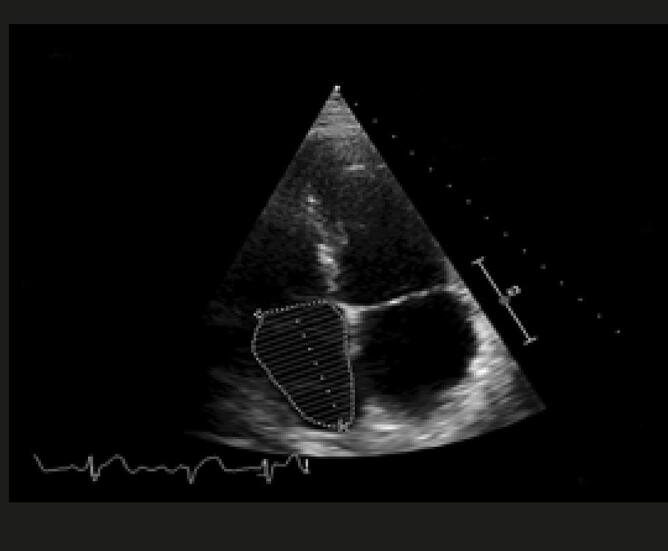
O parâmetro recomendado para avaliar o tamanho do AD é seu volume calculado usando a técnica com plano único do somatório dos discos (2D), no corte apical 4 câmaras realizado adequadamente. VN: Homem 25 ± 7mL/cm ^2 18-32^ Mulher 21 ± 6mL/cm ^2 15-27^
